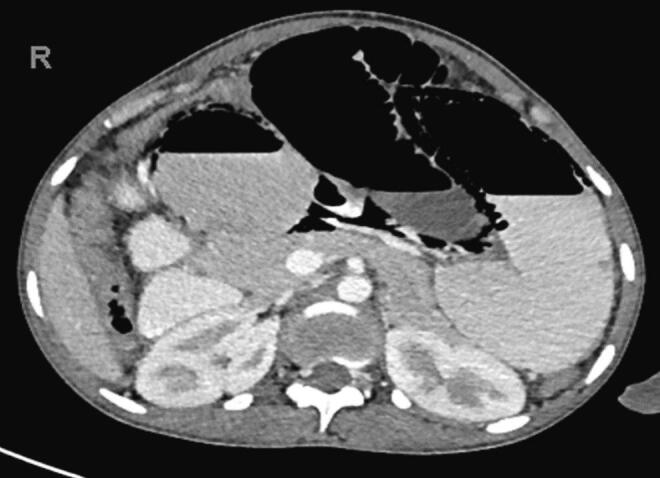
Contrast enhanced CT abdomen showing endometrial free fluid with poor myometrial enhancement. There is also a pelvic transition point.
